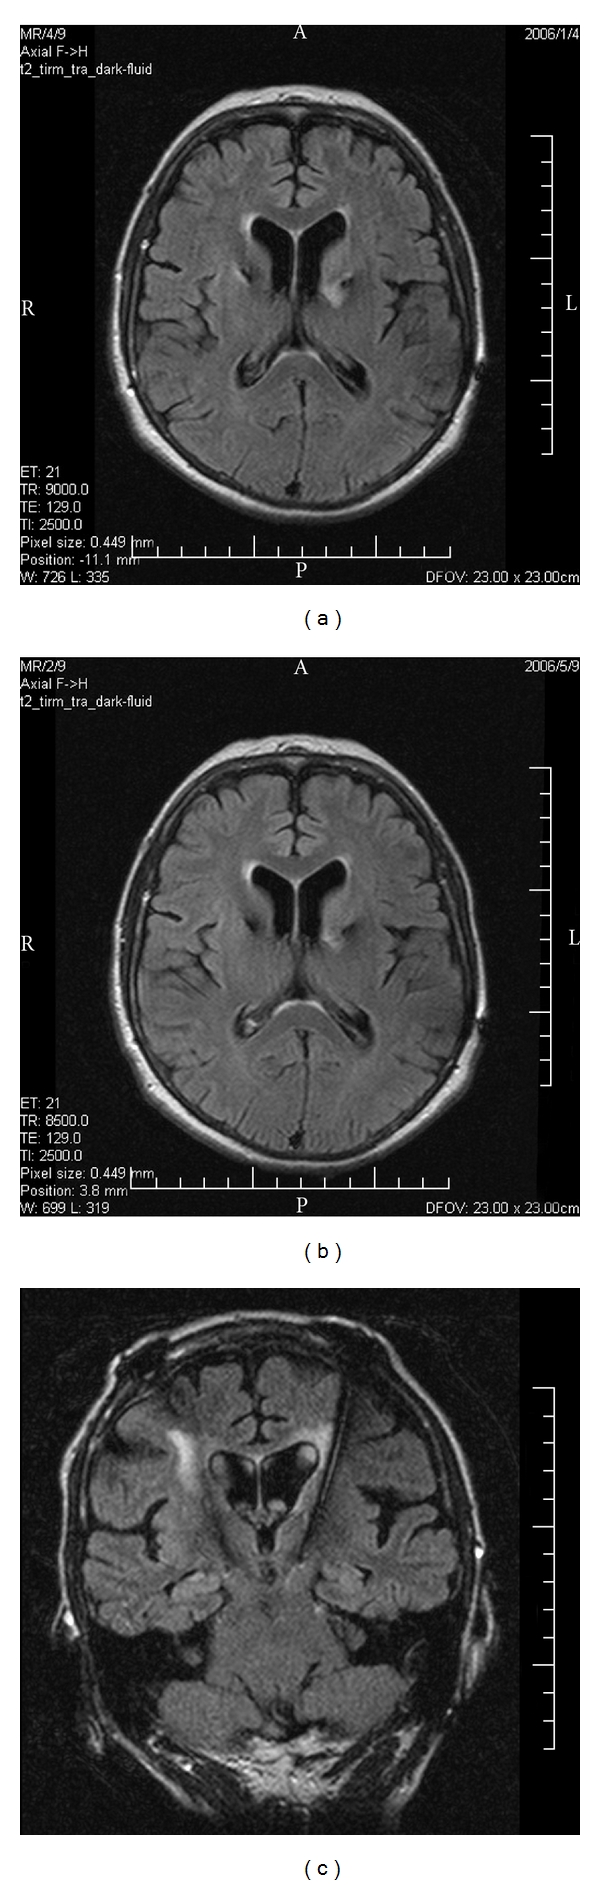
(a) Fluid-attenuated inversion recovery magnetic resonance imaging (FLAIR MRI) in January 2006 shows mild edema and bleeding in the anterior limb of the left internal capsule and left striatum. (b) FLAIR MRI in May 2006 still depicts signal changes along the left electrode trajectory in the internal capsule, medial edge of the lentiform nucleus reaching to the left thalamus. (c) FLAIR MRI in December 2008 demonstrates marked brain atrophy and white matter hyperintensities along the electrode trajectories.
